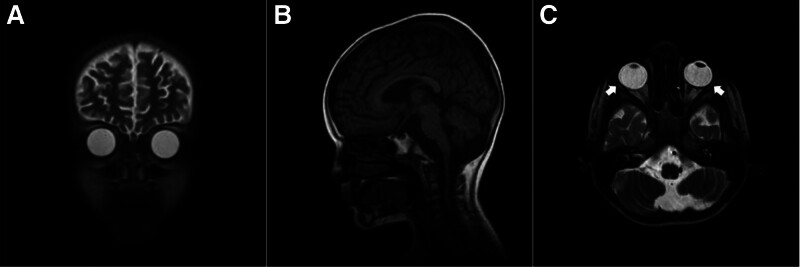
MRI revealing (A) cortical atrophy in the cerebrum, (B) cerebellar atrophy, thinning of the corpus callosum, mega cisterna magna, and (C) bilateral atrophy of lacrimal glands (arrows). MRI = magnetic resonance imaging.
